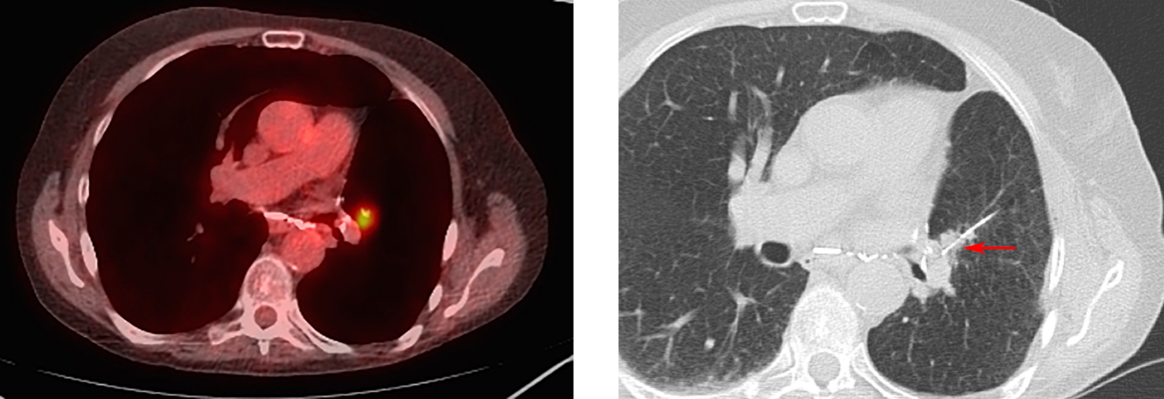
Example of likelihood of adequacy score 1 (low). (A) Fused PET/CT image showing a left lung nodule that though FDG avid is small, deep in emphysematous lung (better seen in b), and adjacent to the pulmonary artery. (B) Arrow points to needle in left lung nodule.
